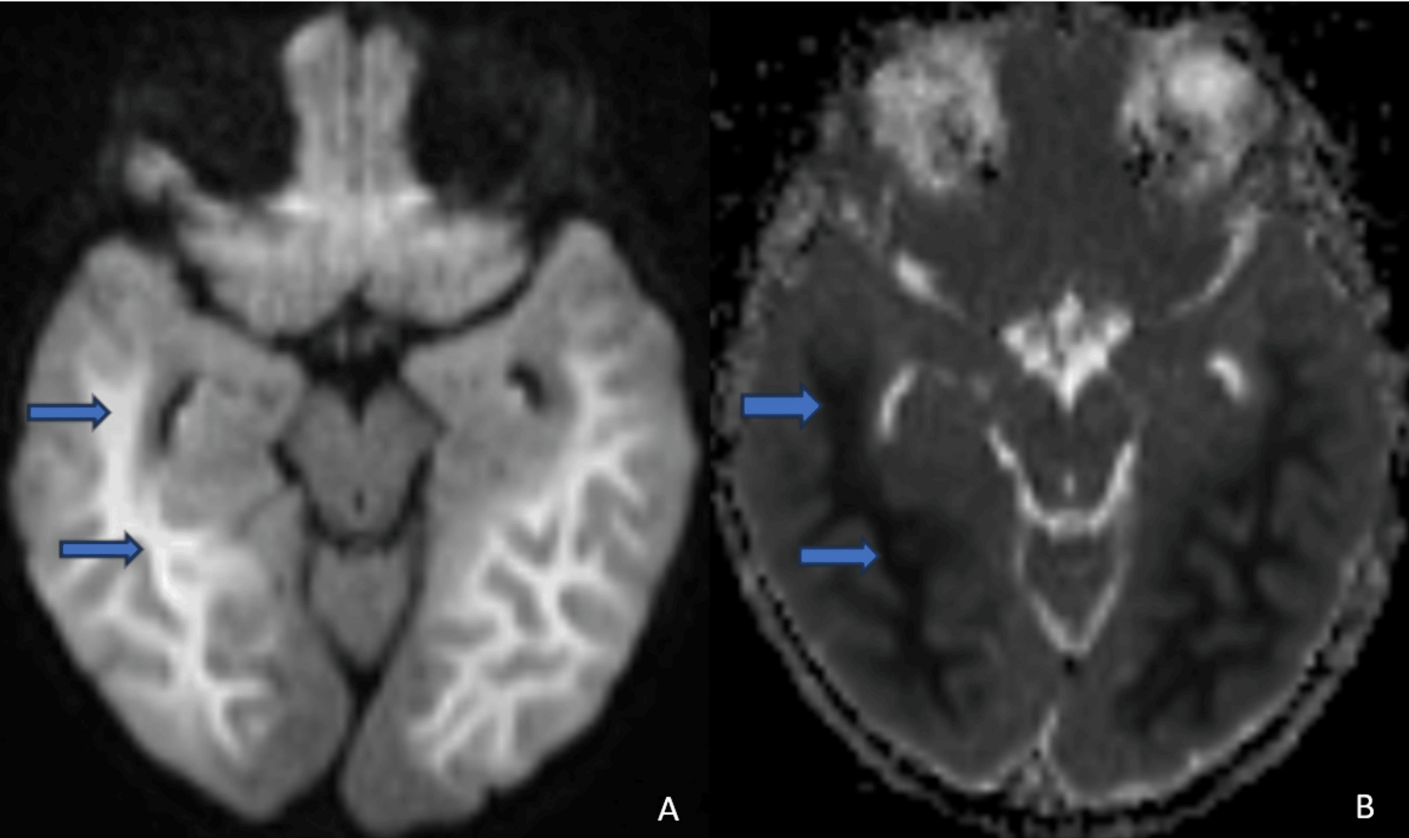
Diffusion weighted images (DWI) and corresponding ADC images show marked diffusion restriction in the form of a high signal on DWI [2A] and a corresponding low signal on ADC [2B] in the subcortical white of bilateral temporal and occipital lobes (blue arrow). ADC: Apparent diffusion coefficient, DWI: Diffusion weighted imaging
